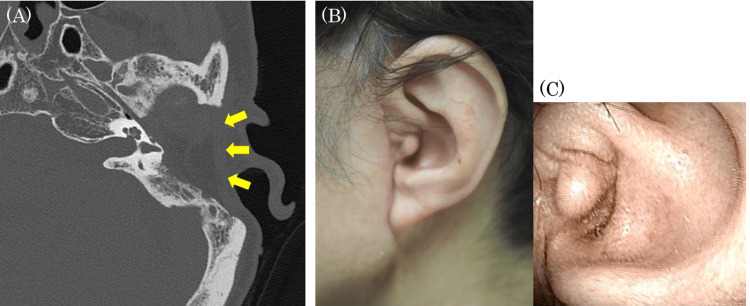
Postoperative findings (A) CT image six months after surgery: filled with a pedicled temporal fascia and abdominal fat; (B) Photograph of the external ear (whole auricle); (C) Photograph of the external ear (closed portion of the external auditory canal)
